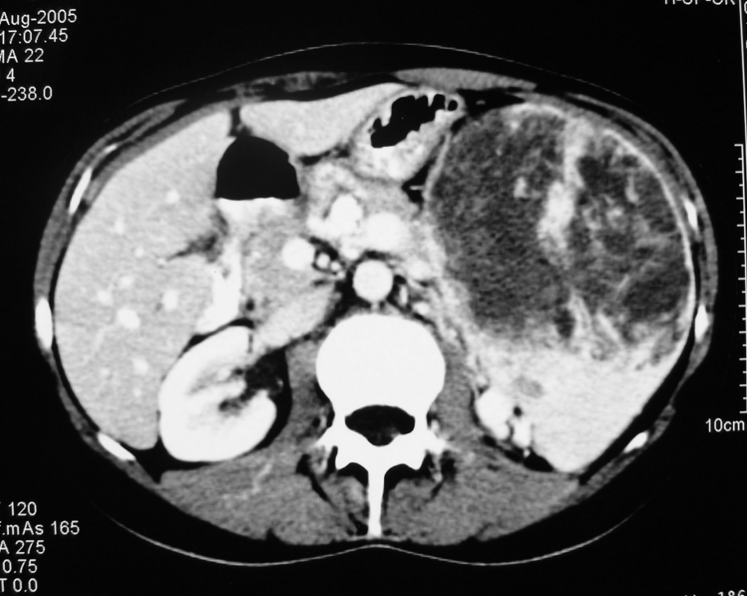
Computed tomography appearance of giant splenic hemangioma and liver hemangiomatosis. Cavernous hemangioma of the spleen and multiple hepatic cavernous hemangiomas displayed in a computed tomography hypodense image after IV contrast injection and peripheral, centripetal enhancement.
